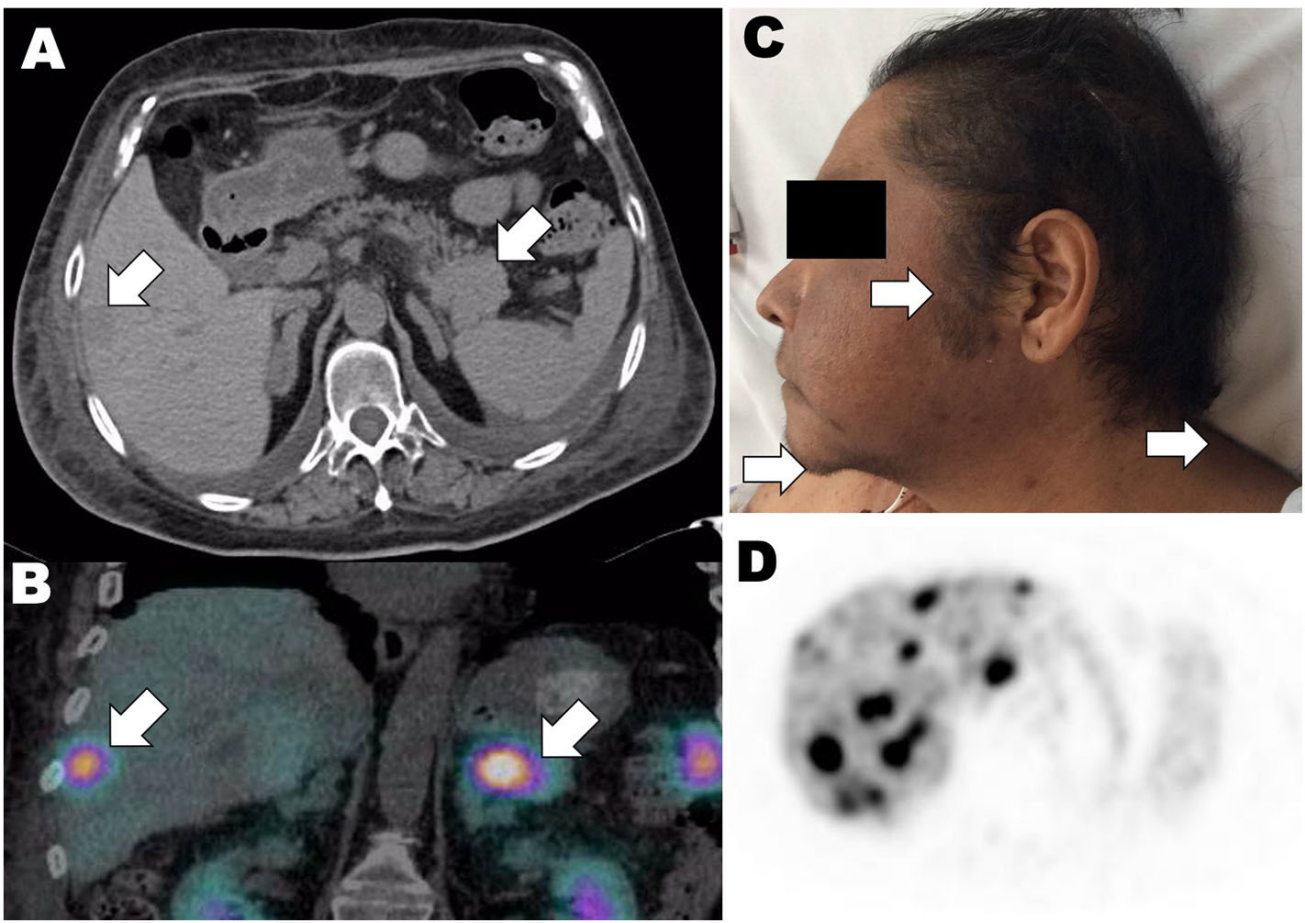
(A) Computerized tomography showing neuroendocrine tumor at the pancreatic tail causing liver metastases (white arrows). (B) Octreotide-single-photon emission computerized tomography (SPECT) study showing hepatic and pancreatic focal lesions with enhanced uptake (white arrows). (C) Clinical picture of the patient remarking the skin hirsutism (white arrows), and hyperpigmentation. (D) 18-Fluorodeoxyglucose (18-FDG) positron emission tomography-computerized tomography (PET-CT) 18 months after diagnosis with persistence of tumor liver metastases (black spots).
